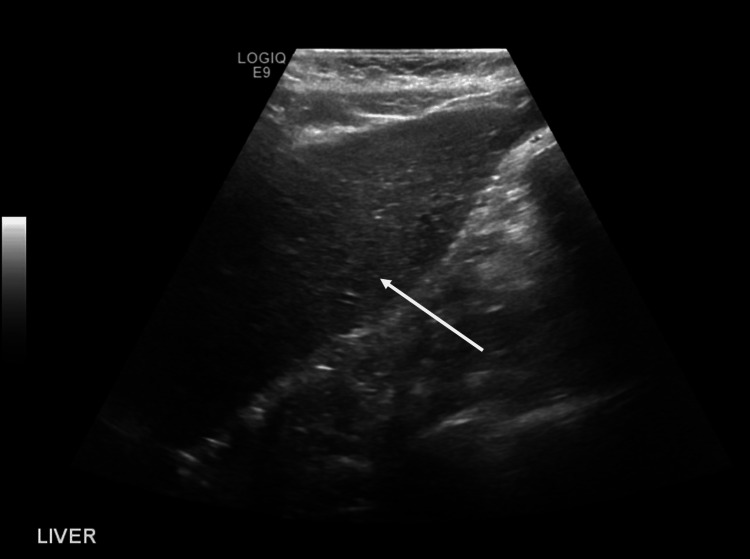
Ultrasound image of the patient's liver. The liver is normal in size measures 9.9 cm, with normal homogeneous parenchymal echogenicity and smooth surface outline. No obvious focal hepatic lesion could be seen.
